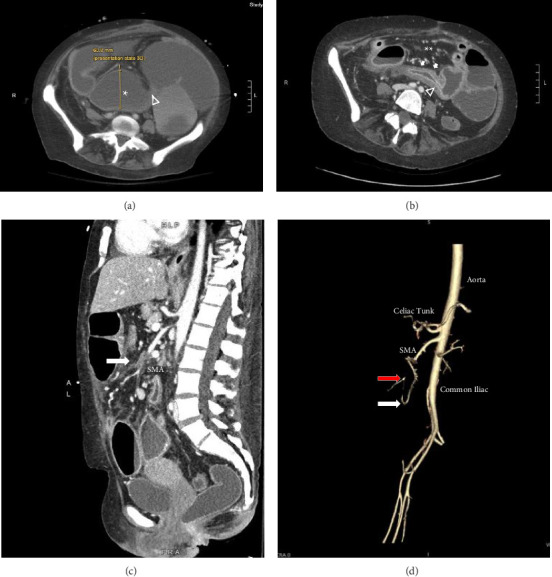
Computed tomography (CT) images of multifocal long-segment ileal and jejunal stricture secondary to superior mesenteric artery (SMA) thrombus following ileocolic resection: (a) axial CT demonstrating an ileal stricture (arrowhead) with proximal small bowel dilation (^∗^) to 60.8 mm. (b) Axial CT enterography demonstrating an ileal stricture (arrowhead) with bowel wall thickening and mural hyperenhancement (solid arrow) with adjacent vasa recta engorgement (^∗∗^). (c) Sagittal CT mesentery with (d) 3D volume-rendered imaging demonstrating the proximal point of SMA cutoff (RED arrow) and distally reconstituted branches (white arrow).
